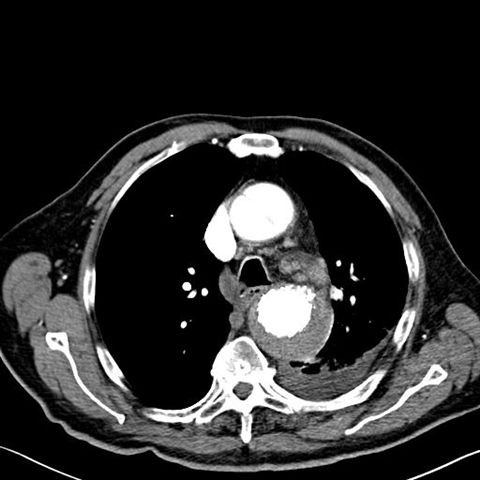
Computed tomographic showing the periaortic hematoma.
